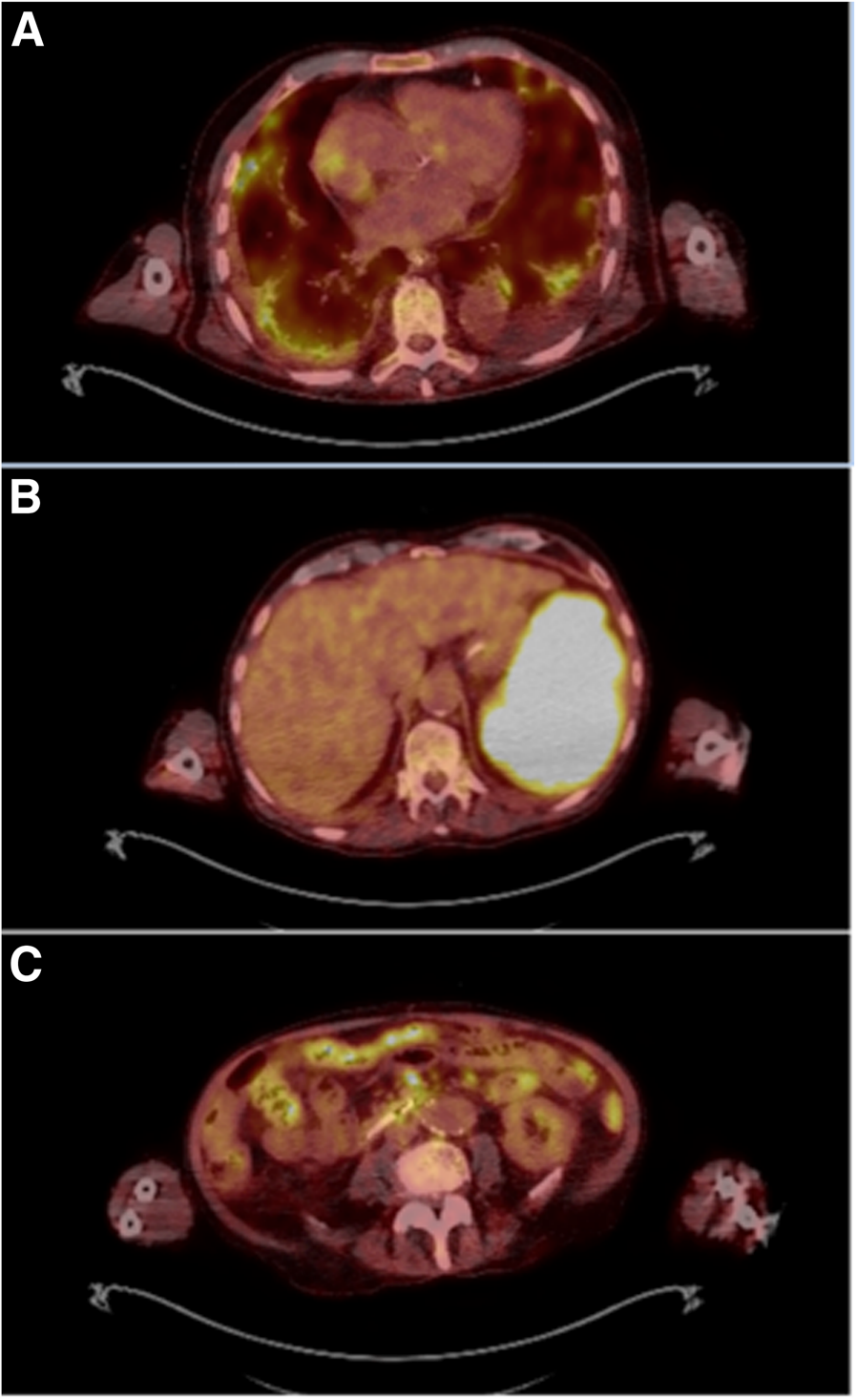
Transverse images of ^18^ F-FDG PET-CT showing intense uptake of FDG into (a) pleural base, (b) the enlarged spleen (18 cm) and (c) colon and peritoneal lymph nodes
